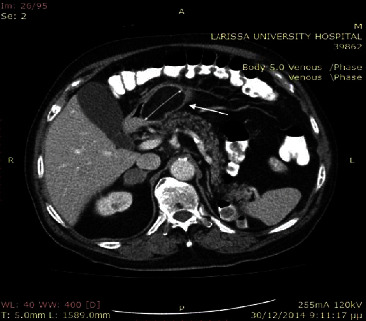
The computed tomography revealed a 5.6 cm × 3.5 cm hypodense soft-tissue mass in the antrum of the stomach (white arrows).
